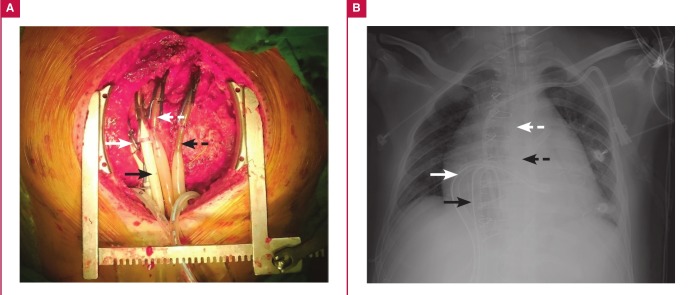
(A) Photograph of the cardiac operation and (B) a chest X-ray showing the left heart venous drainage tube (white arrow), the arterial perfusion tube (white dotted arrow), the right heart venous drainage tube (black arrow), and the arterial perfusion tube (black dotted arrow).
